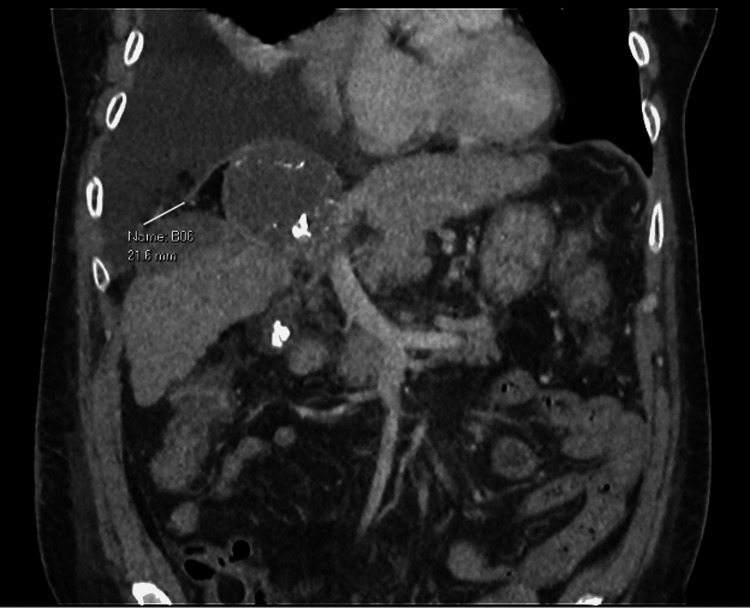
Surveillance CT liver done in October 2024 demonstrating a 2.2 cm right diaphragmatic defect (adjacent to the treated segment VIII/V lesion), with herniation of fat into the right lower hemithorax, with concerns of a possible pleuro-peritoneal fistula.
